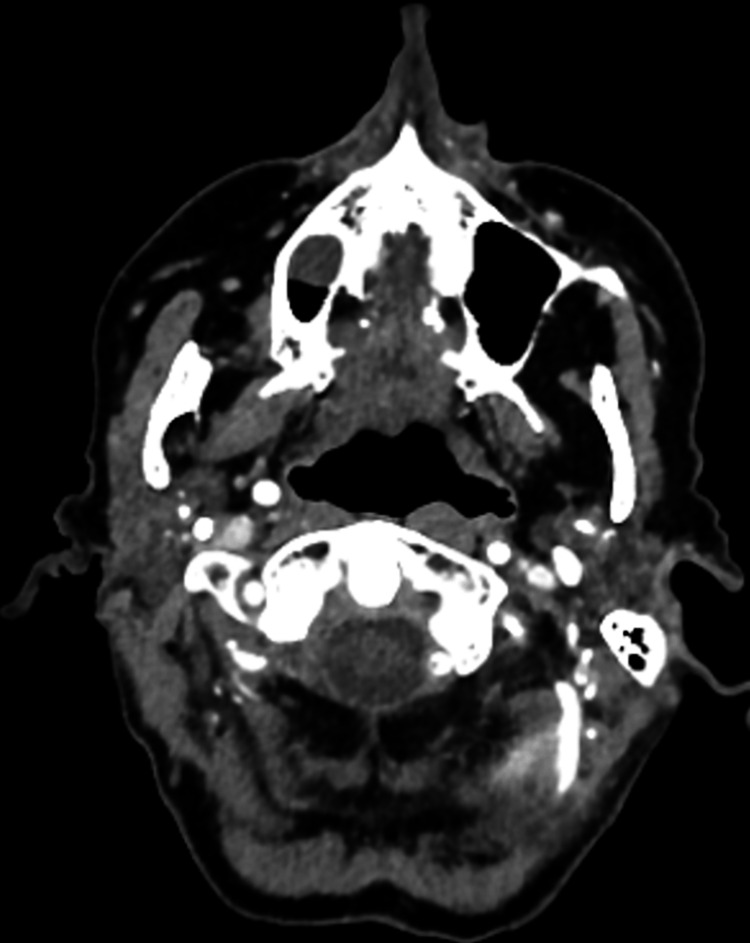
An axial CT head angiogram demonstrating thrombus in both distal vertebral arteries.
